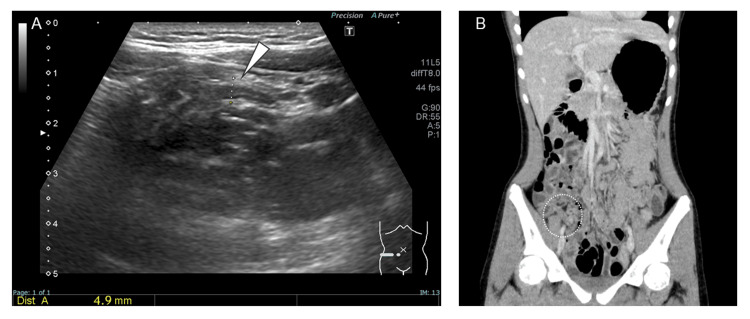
Appendiceal imaging findings A: Abdominal ultrasonography showing a longitudinal view of the appendix, indicated by the white arrowhead. The maximum outer diameter is 4.9 mm, which is within the normal range (<6 mm). No wall thickening is observed. B: Contrast-enhanced abdominal computed tomography image. The appendix is located within the white dotted circle. There is no evidence of appendiceal enlargement, wall thickening, or periappendiceal inflammation.
